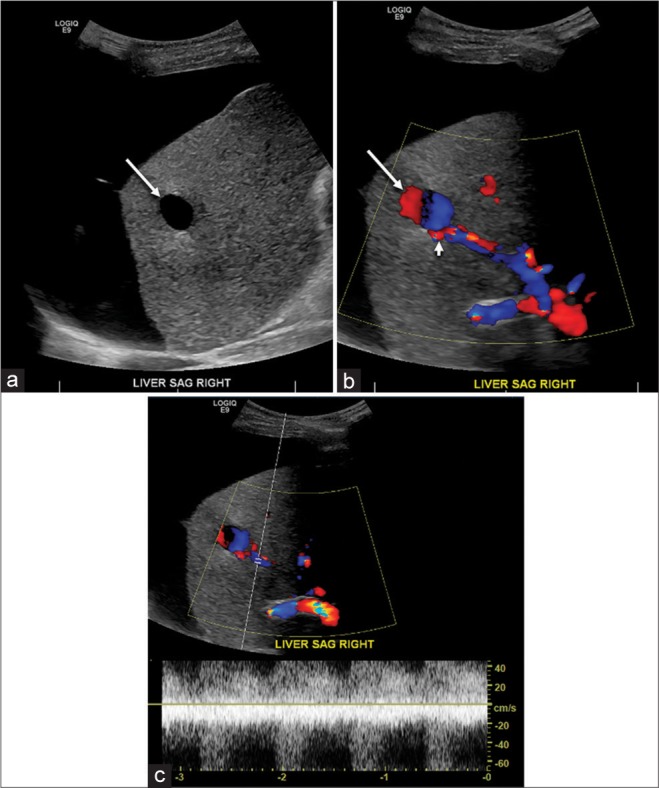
(a) Sagittal gray-scale image of the liver demonstrates a 1.5-cm anechoic lesion in the right hepatic lobe (arrow) with minimal through transmission. Also note moderate volume simple perihepatic ascites. (b) Sagittal color Doppler image of the liver shows the anechoic lesion in the right hepatic lobe with yin-yang pattern of internal flow (arrows) and a vascularized neck (arrowhead).(c) Sagittal color Doppler image of the right hepatic lobe lesion demonstrates a high-velocity low-resistance spectral waveform in the neck of the lesion.
